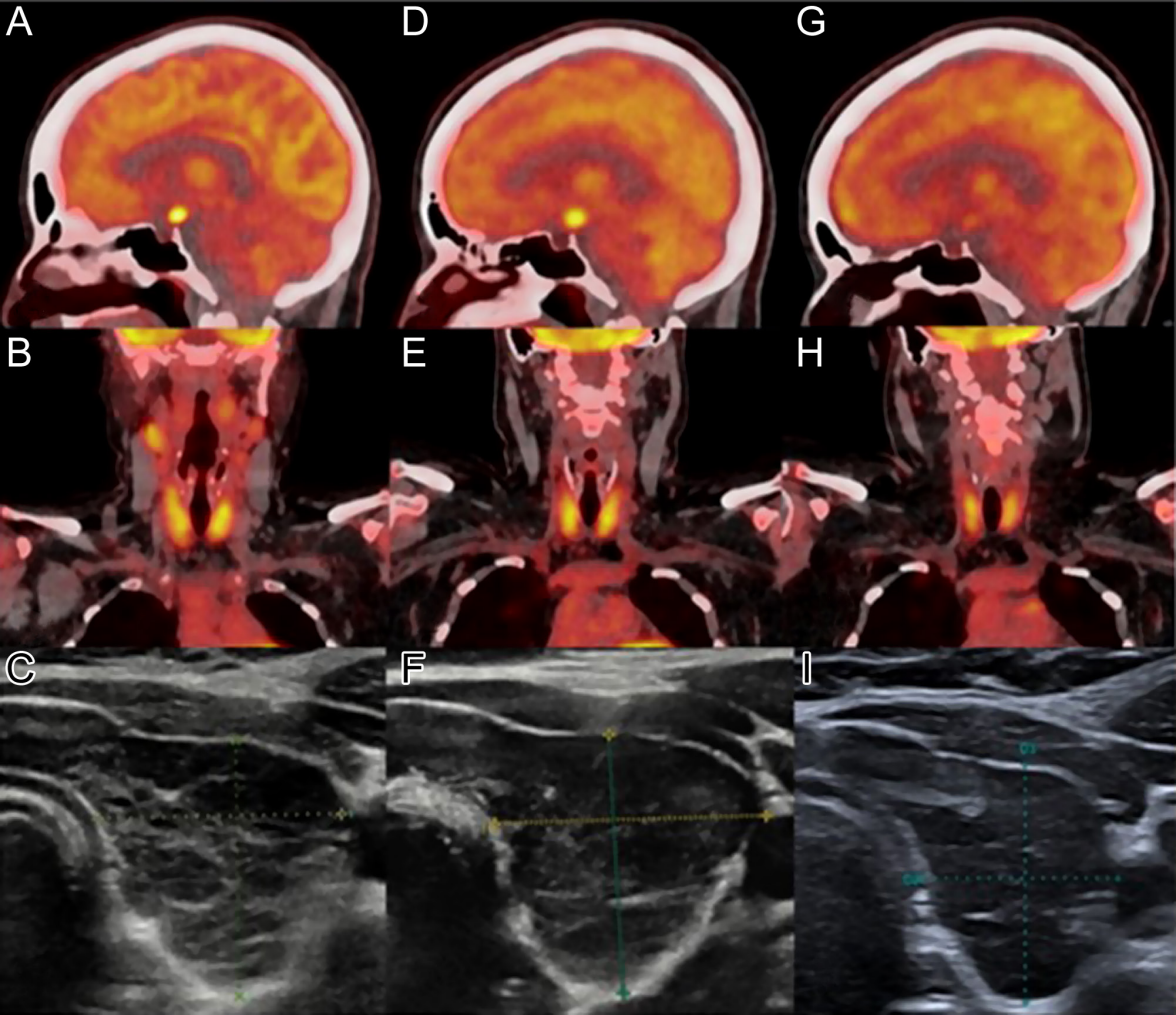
Whole-body FDG-PET/CT and thyroid ultrasound. Sagittal views (A and D) of FDG-PET/CT demonstrating intense FDG uptake of the suprasellar tumor decreasing after cladribine treatment (G). Coronal views (B, E) show moderate, diffuse thyroid hypermetabolism, persisting after treatment (H). Transverse image of the left thyroid lobe (C) upon presentation (thyroid volume 19 mL), on follow-up before specific diagnosis (F) and treatment (thyroid volume 26.7 mL), and after cladribine treatment (I) (thyroid volume 16.2 mL).
